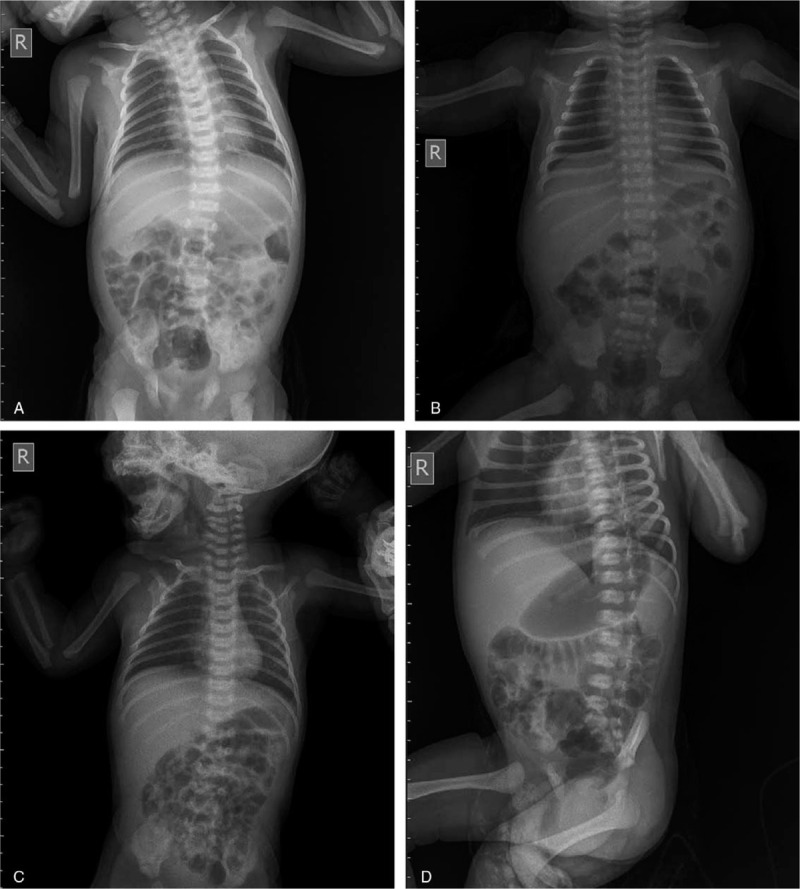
(A) Chest X-ray of patient 1 on admission, showing normal radiologic appearance of lungs. (B) Chest X-ray of patient 2 on admission, showing normal radiologic appearance of lungs. (C) Chest X-ray of patient 3 on admission – medium degree of hilar parenchymal infiltration and a slight infiltration of the visceral pleura. (D) Chest X-ray of patient 3 on discharge, normal radiologic appearance of lungs, with no pathologic signs.
